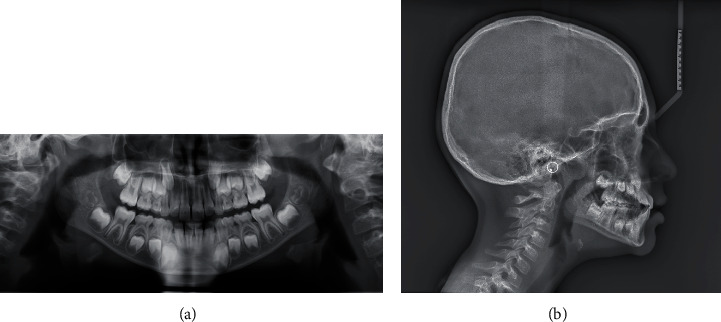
Post-treatment radiographic records: (a) orthopantomogram; and (b) lateral cephalogram.
